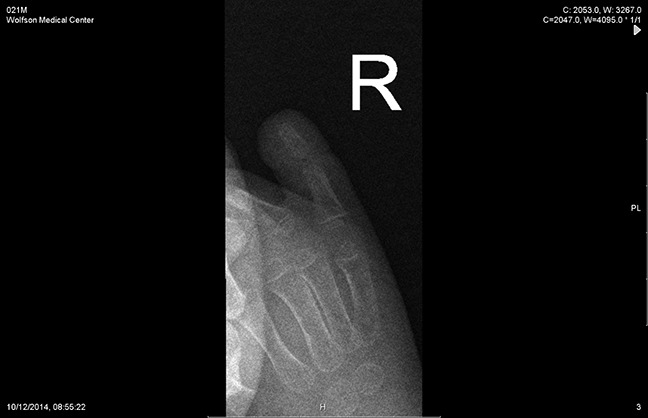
Radiograph of the distal phalanx amputation, oblique view
